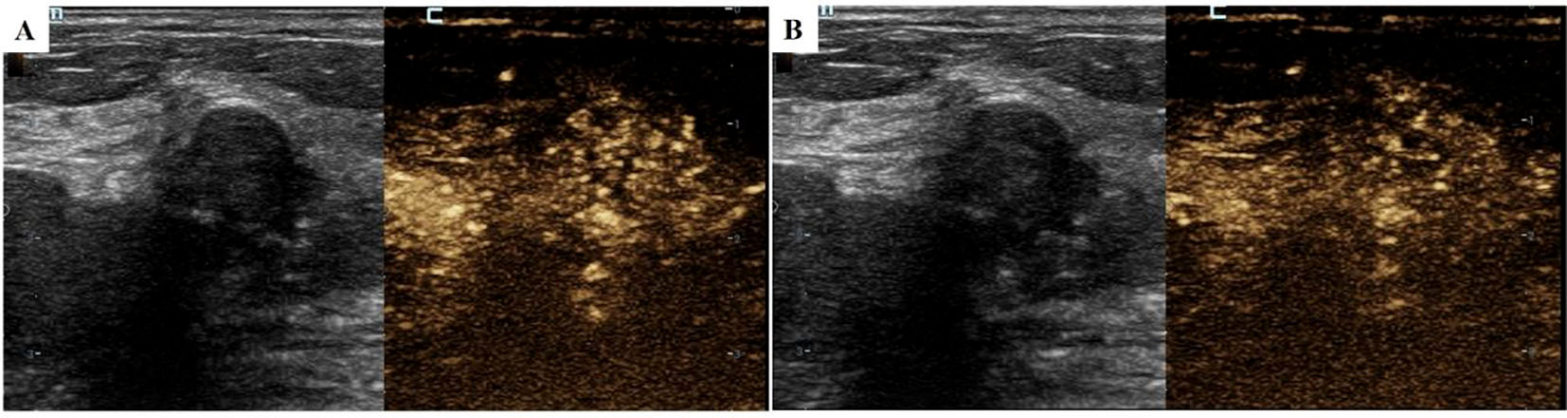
CEUS images of the tumor. (A) CEUS arterial phase of right breast nodule (this image was taken on June 11, 2024; the image shows the situation 20 seconds after the contrast agent was injected); (B) CEUS venous phase of right breast nodule (this image was taken on June 11, 2024; the image shows the situation 50 seconds after the contrast agent was injected); US, ultrasound; CEUS, contrast-enhanced ultrasound.
